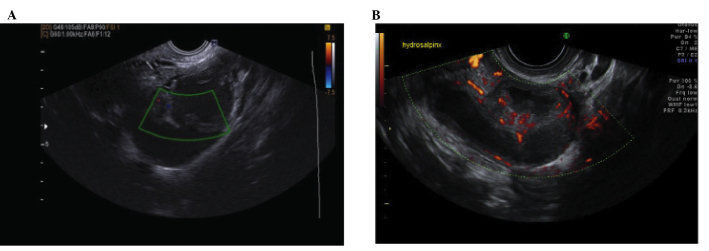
Acute salpingitis demonstrating incomplete septae and thick walls. (A) An example of increased vascularity in an incomplete septum using color Doppler TVS. (B) Another example using power Doppler TVS.
